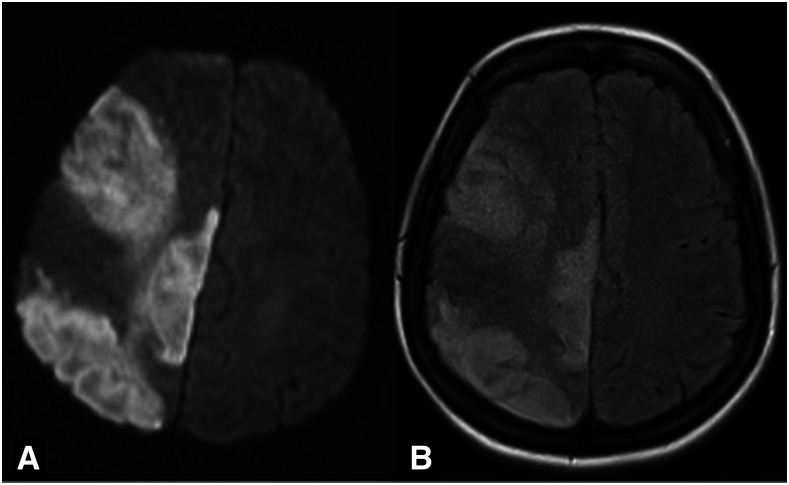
Magnetic resonance imaging (MRI). (A) shows confluent diffusion-weighted imaging hyperintensities of the right frontal temporal lobe, right temporal parietal, and frontal lobe with panel (B) MRI showing corresponding fluid-attenuated inversion recovery hyperintensities.
